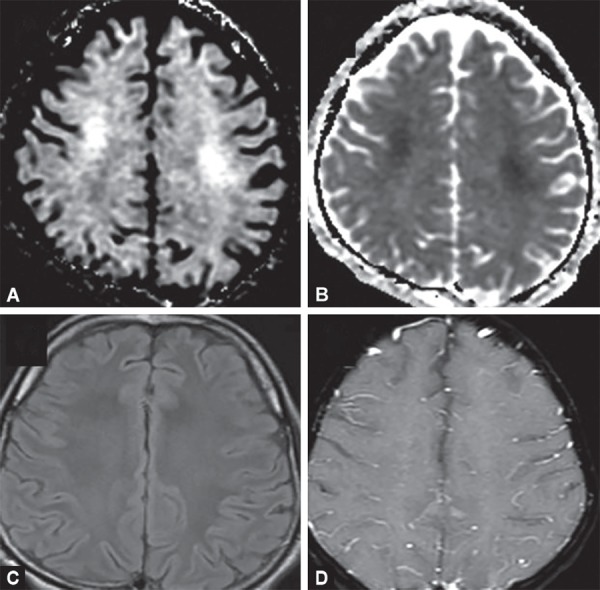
Methotrexate toxicity. Symmetric diffusion restriction in high frontal white mater (A and B) is the earliest change on imaging, where as FLAIR (C)and contrast images (D) are normal
